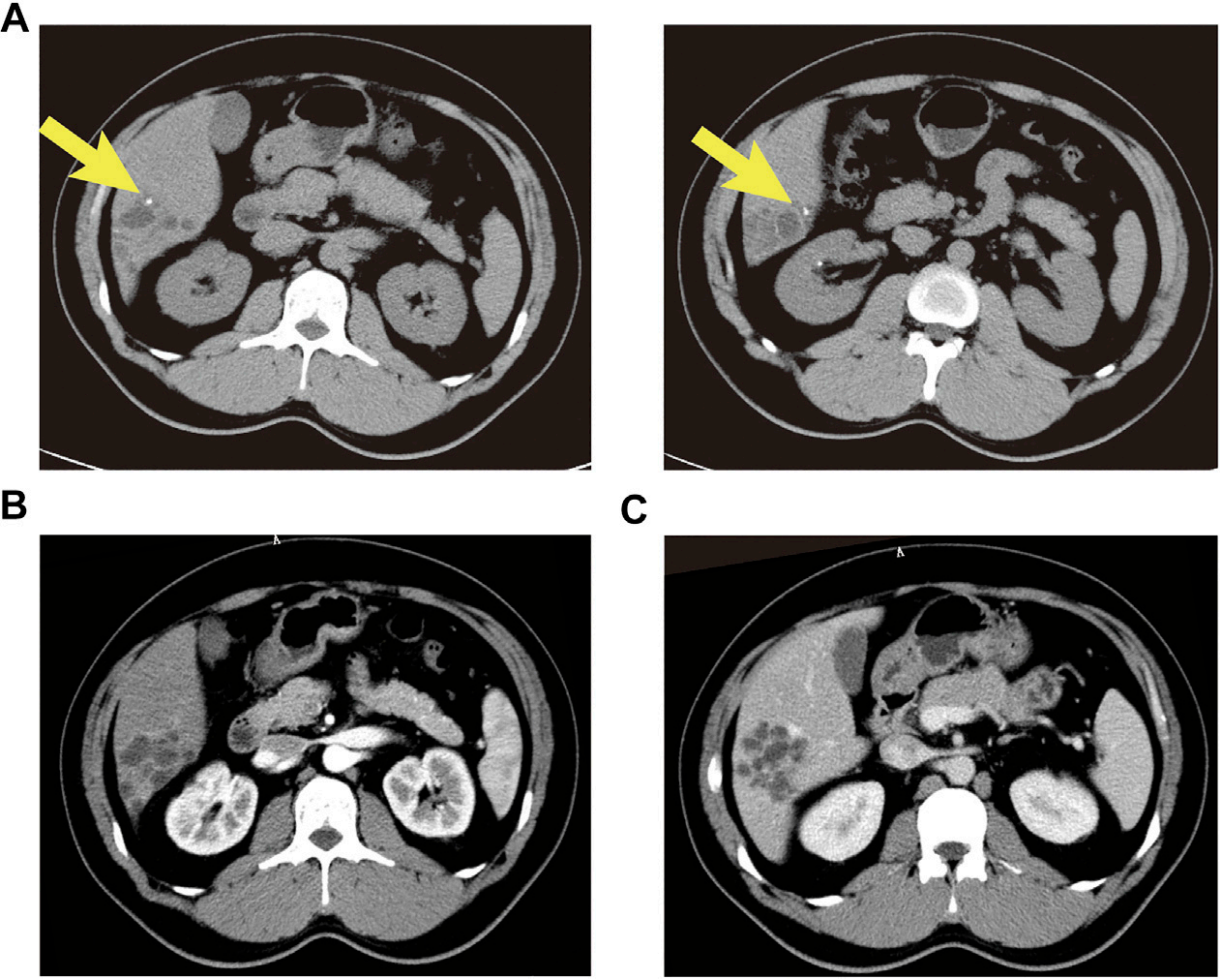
Abdominal contrast enhanced CT showed an intrahepatic multicystic mass in the segment VI of the liver. (A) Calcification was observed in dilated ducts (plain phase arrow); (B) The enhancement of the septa and peripheral wall within the cystic lesion (arterial phase); (C) The lesion showed a low-density honeycomb-like appearance (venous phase).
